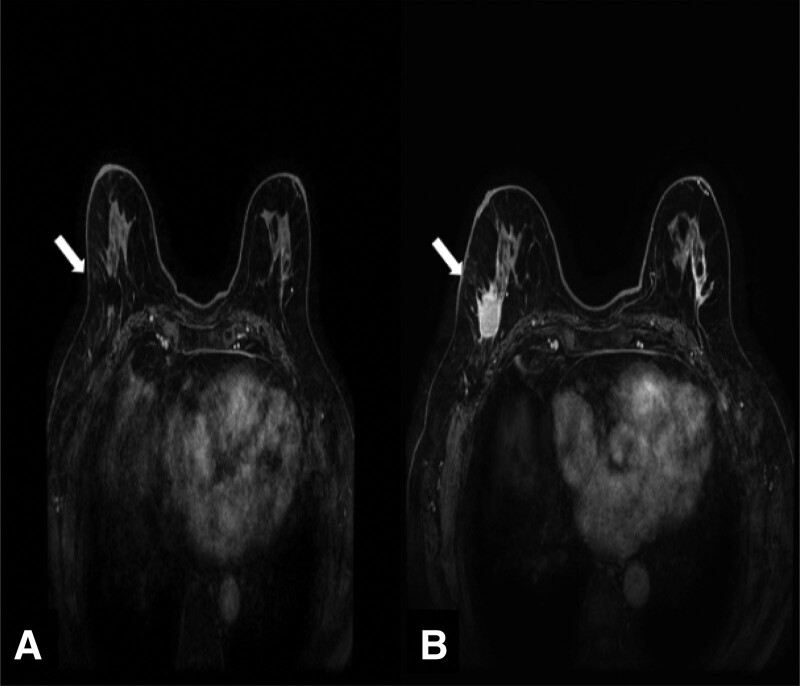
Breast magnetic resonance imaging (MRI) shows (A) an almost complete response to neoadjuvant therapy (18 mm). (B) Initial diagnosis of right breast cancer (46 mm).
